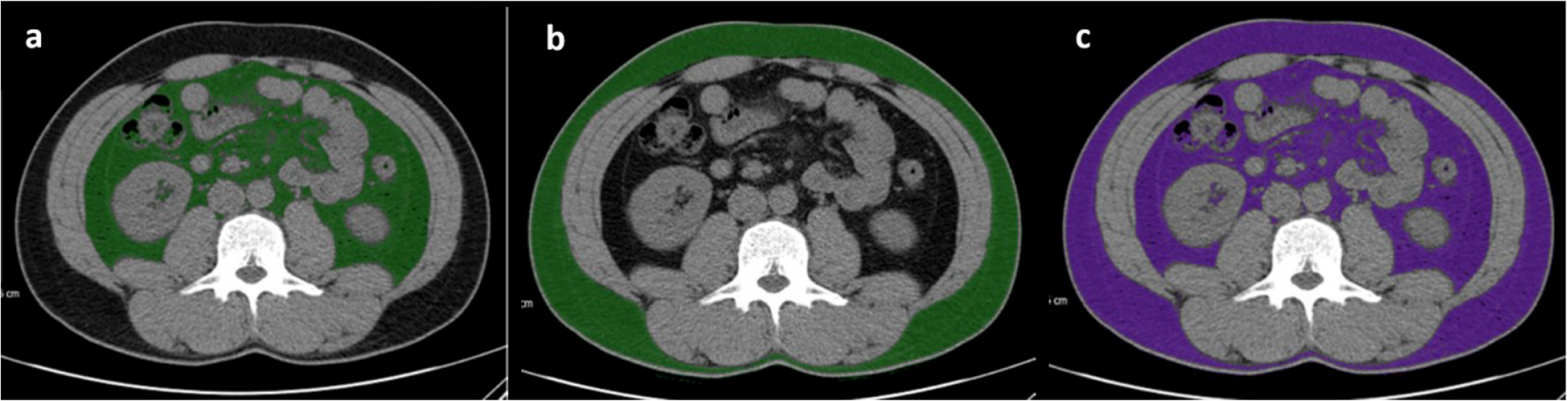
Computed tomography (CT) analysis of visceral and subcutaneous adipose tissue. Measurement of a visceral adipose tissue (VAT), b subcutaneous adipose tissue (SAT), and c total adipose tissue (TAT) by CT analysis software for a representative patient
